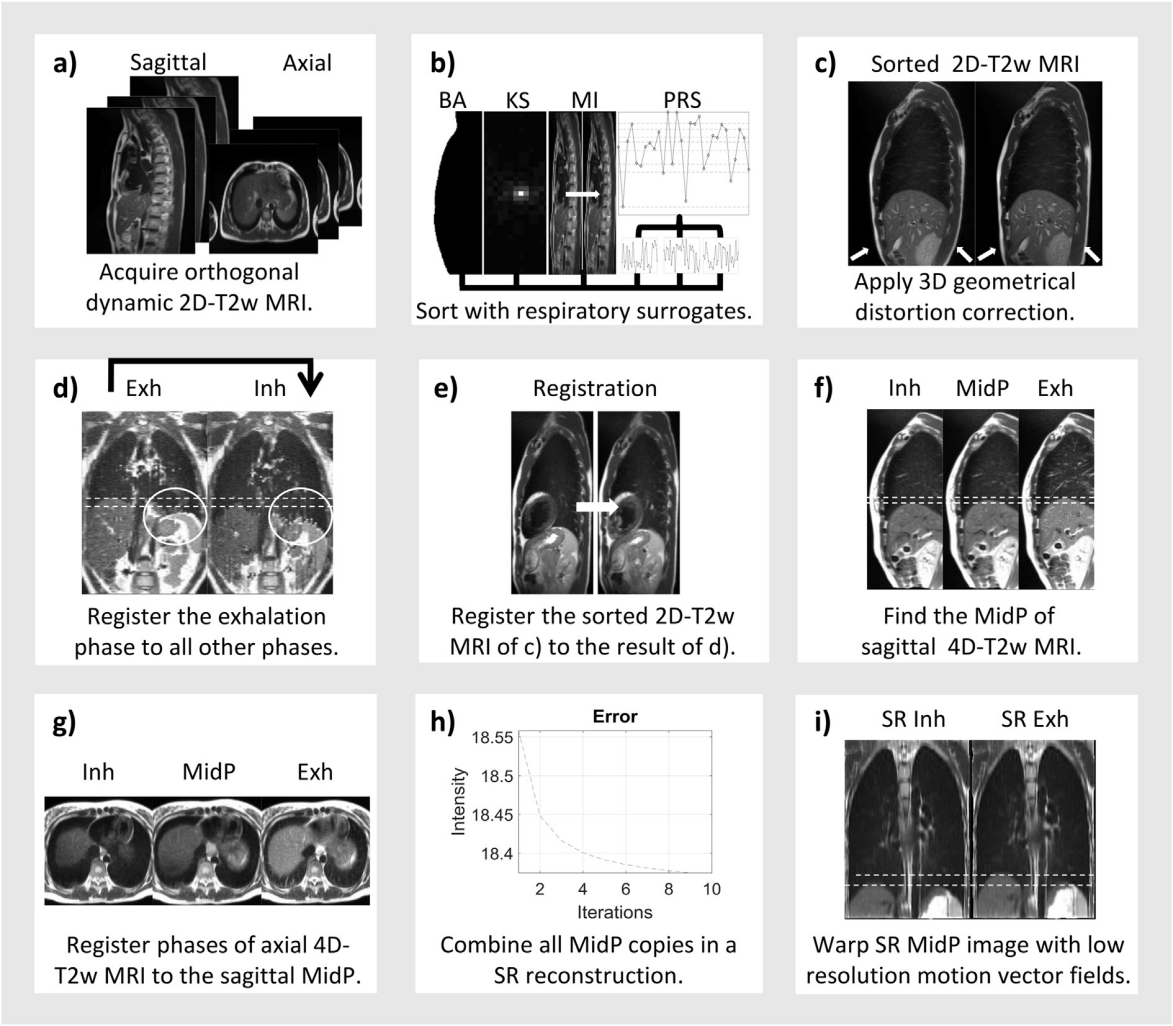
(a) Axial and sagittal 2D-T2w MRI are continuously acquired, (b) retrospectively sorted by the principle respiratory surrogate (PRS), which combines body area (BA), artificial k-space (KS) and mutual information (MI), and (c) distortion corrected. (d) For both axial and sagittal 4D-T2w MRI, stitching and binning artefacts, visible within the white circle, are reduced by registering the exhalation phase to all other respiratory phases. (e) Sorted images in (c) are corrected by registering to the results of (d). (f) Each phase of the sagittal 4D-T2w image of (e) is warped to midposition (MidP). (g) Each phase of the axial 4D-T2w image in (e) is registered to the sagittal MidP. (h) Super-resolution (SR) reconstruction is performed using the sagittal and axial MidP copies. (i) SR 4D-T2w MRI is obtained by warping the SR MidP image with the low-resolution motion vector fields calculated from the corrected sagittal 4D-T2w MRI in (f).
